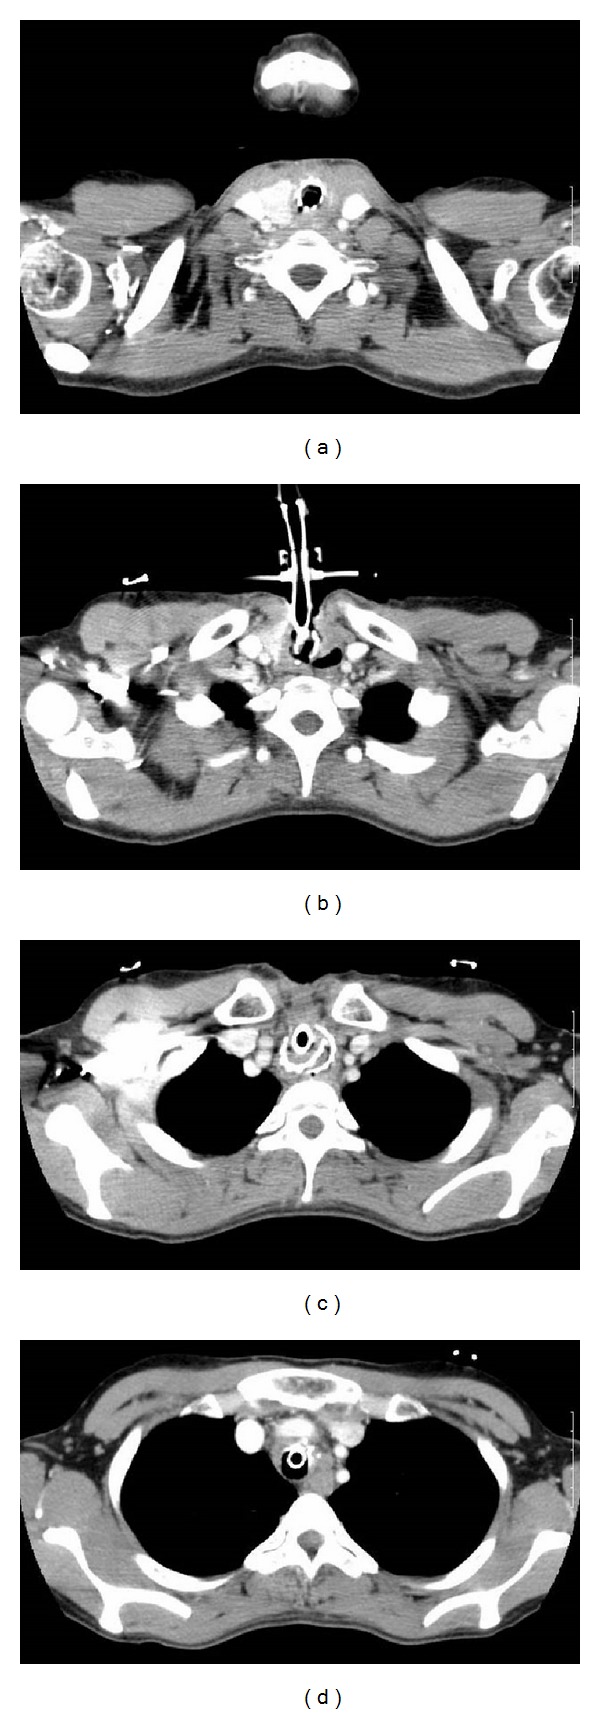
Computed tomography scans. (a) The stent was inserted proximally to the tracheotomy. (b) The tracheotomy was shown on CT. (c) The part of the destroyed stent that was distal from the tracheotomy was not circular. (d) The lower end of the stent was shown on the CT scan.
